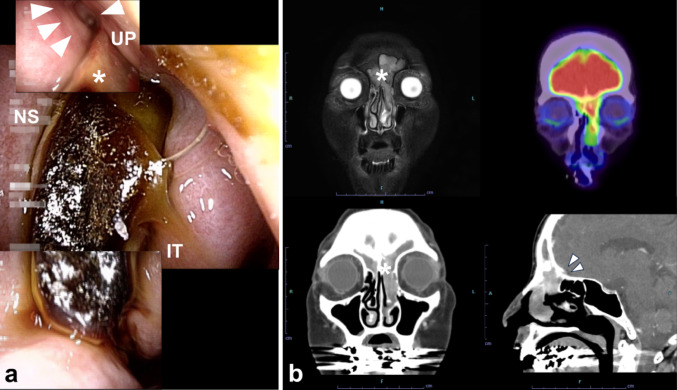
Nasal endoscopy (a), MRI, CT, and PET/CT (b) at initial presentation
